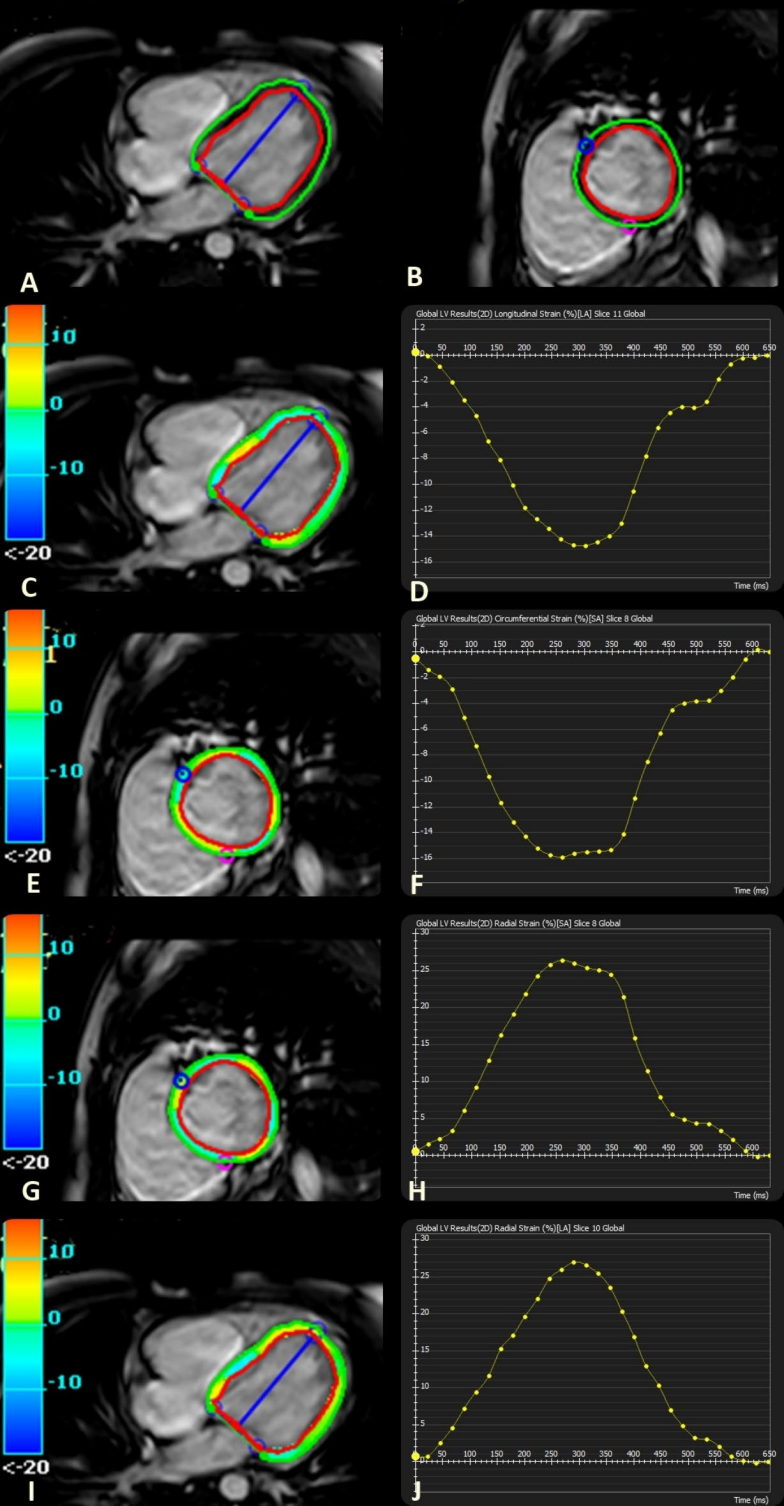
Cardiac MRI tissue tracking strain analysis in a girl with Turner syndrome. (A, B) Long- and short-axes cine SSFP images with tracings of endo- and epicardial left ventricular contours. (C, D) Color map and strain-time curve of global longitudinal strain (GLS). (E, F) Color map and strain-time curve of global circumferential strain (GCS). (G, H) Color map and strain-time curve of global radial strain short axis (GRS SAX). (I, J) Color map and strain-time curve of global radial strain long axis (GRS LAX).
